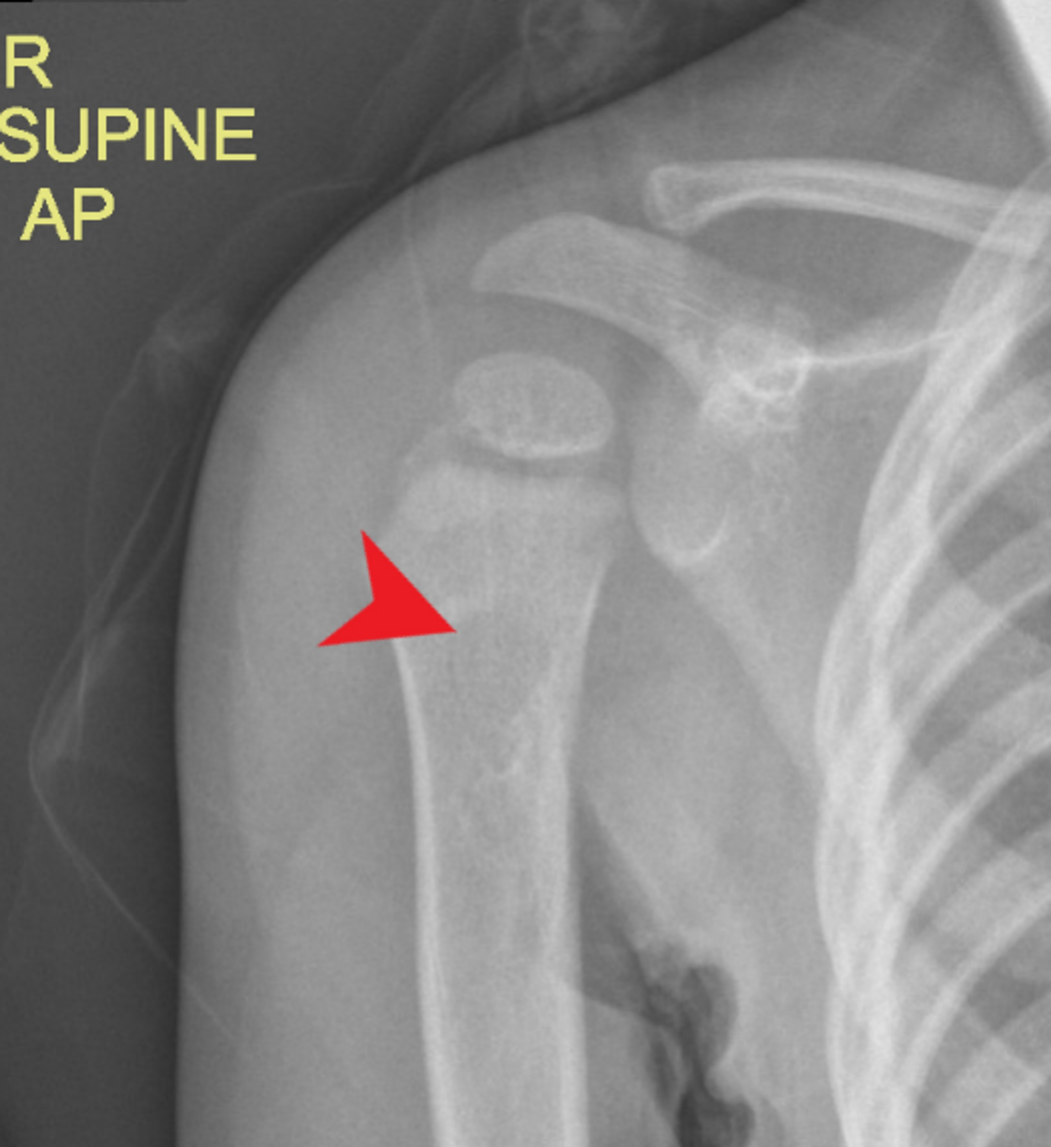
X-ray shows healing of the osteolytic lesion without any periosteal reaction detected.
